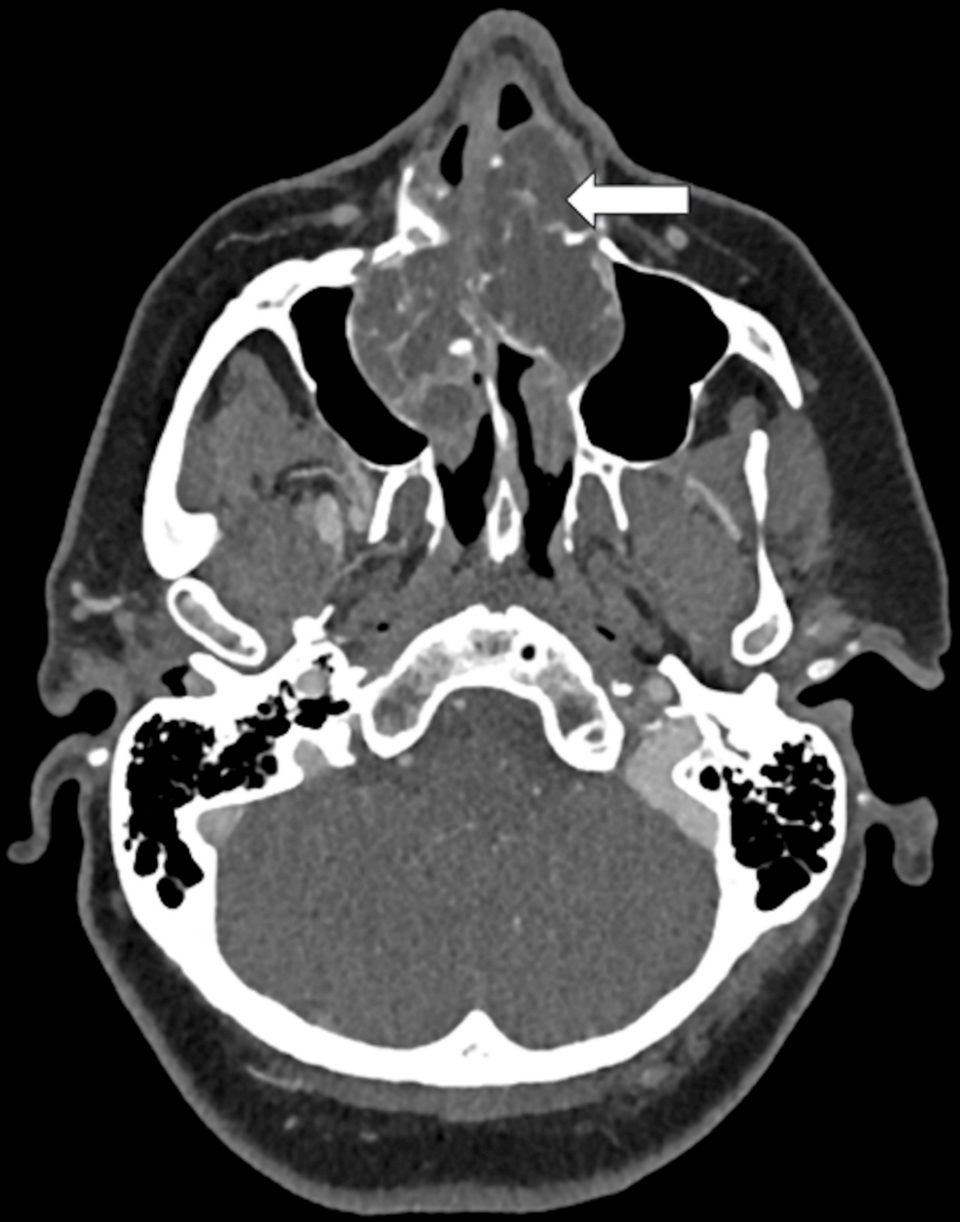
Axial contrast-enhanced CT of sinonasal chondrosarcoma The image shows a large lobulated soft-tissue mass centered on the anterior-inferior nasal septum, extending into both maxillary sinuses and the nasal floor (white arrow). The lesion exhibits internal arciform calcifications typical of a chondroid matrix and causes remodeling of adjacent bony structures CT: computed tomography
